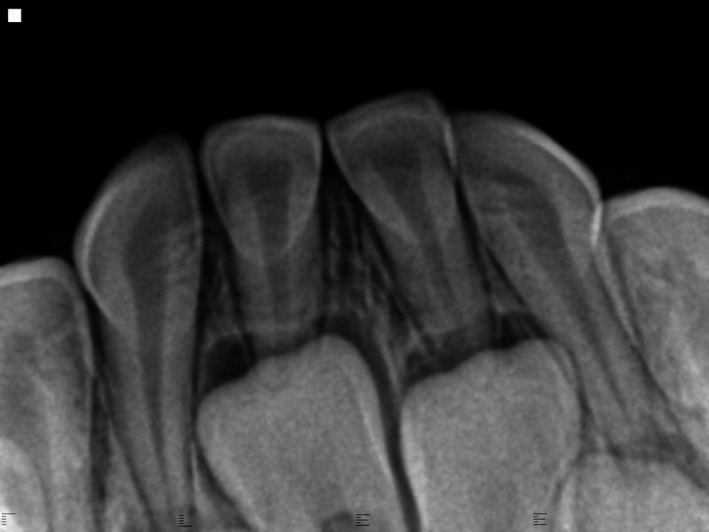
Preoperative radiograph of the region showing slight radiolucency mesial to tooth #R in the region of the gingival lesion
